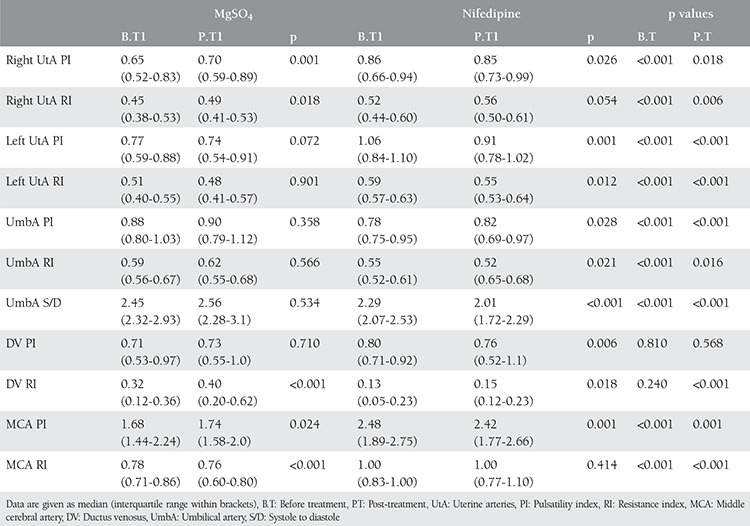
Doppler indexes in groups before treatment and at 48 hours post-treatment
